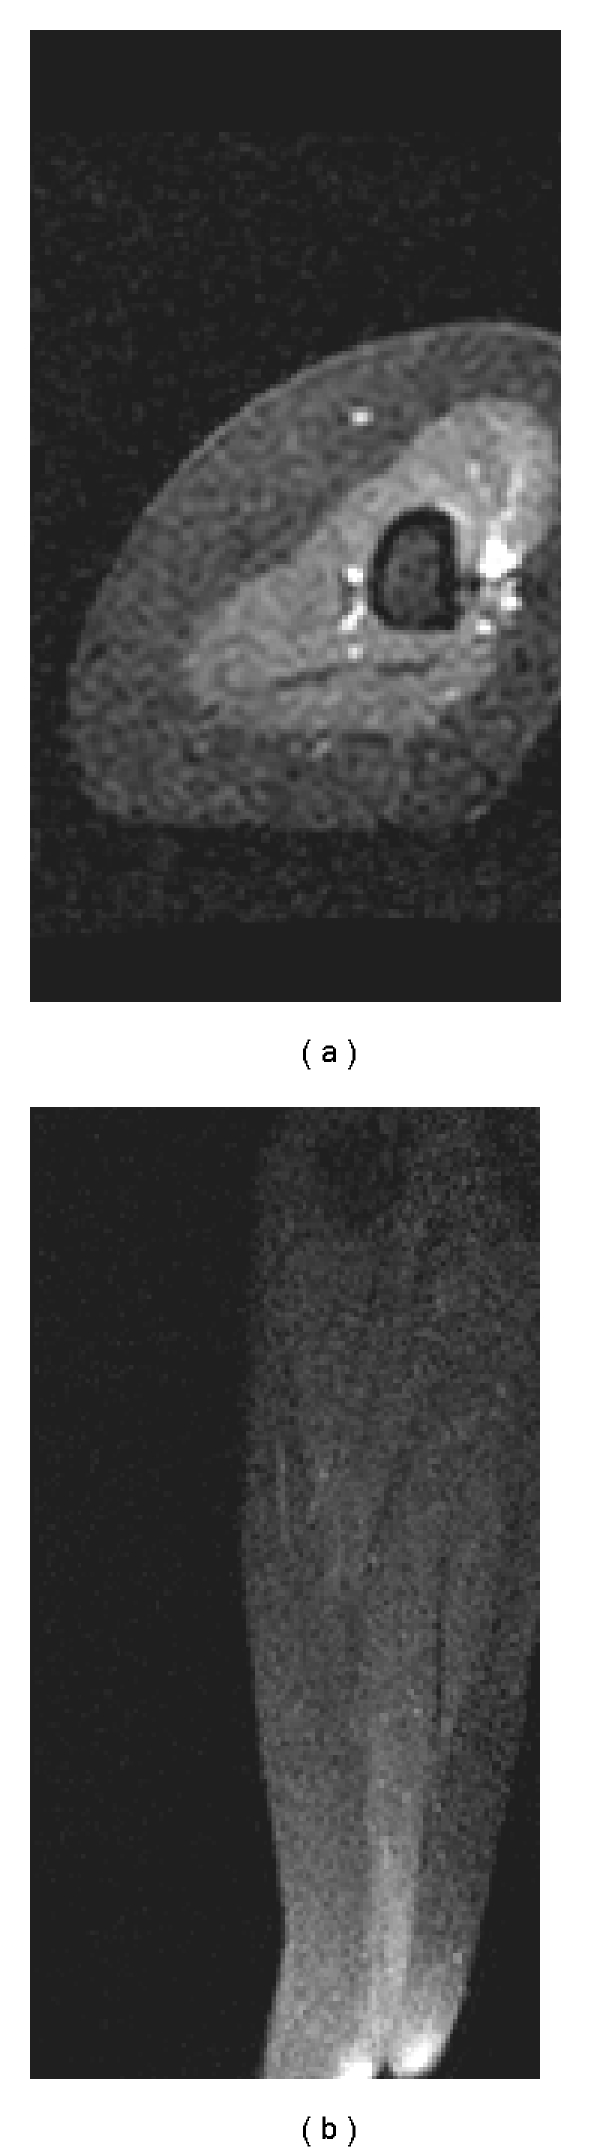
Posttreatment axial (a) and sagittal (b) postgadolinium contrast MRI of the right upper arm demonstrating resolution after a course of IFRT to 40 Gy.
